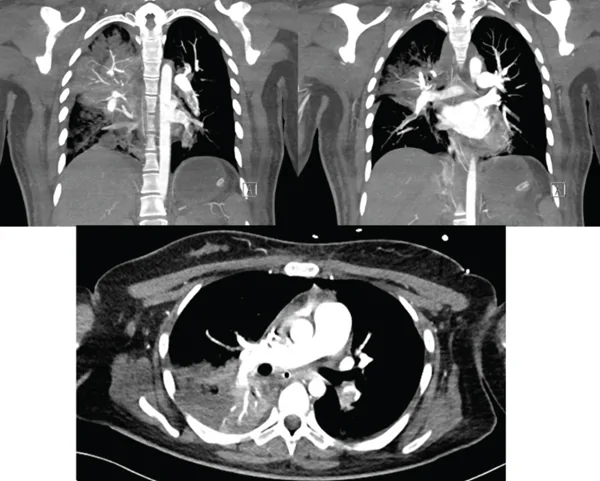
Admission chest CT angiography.
